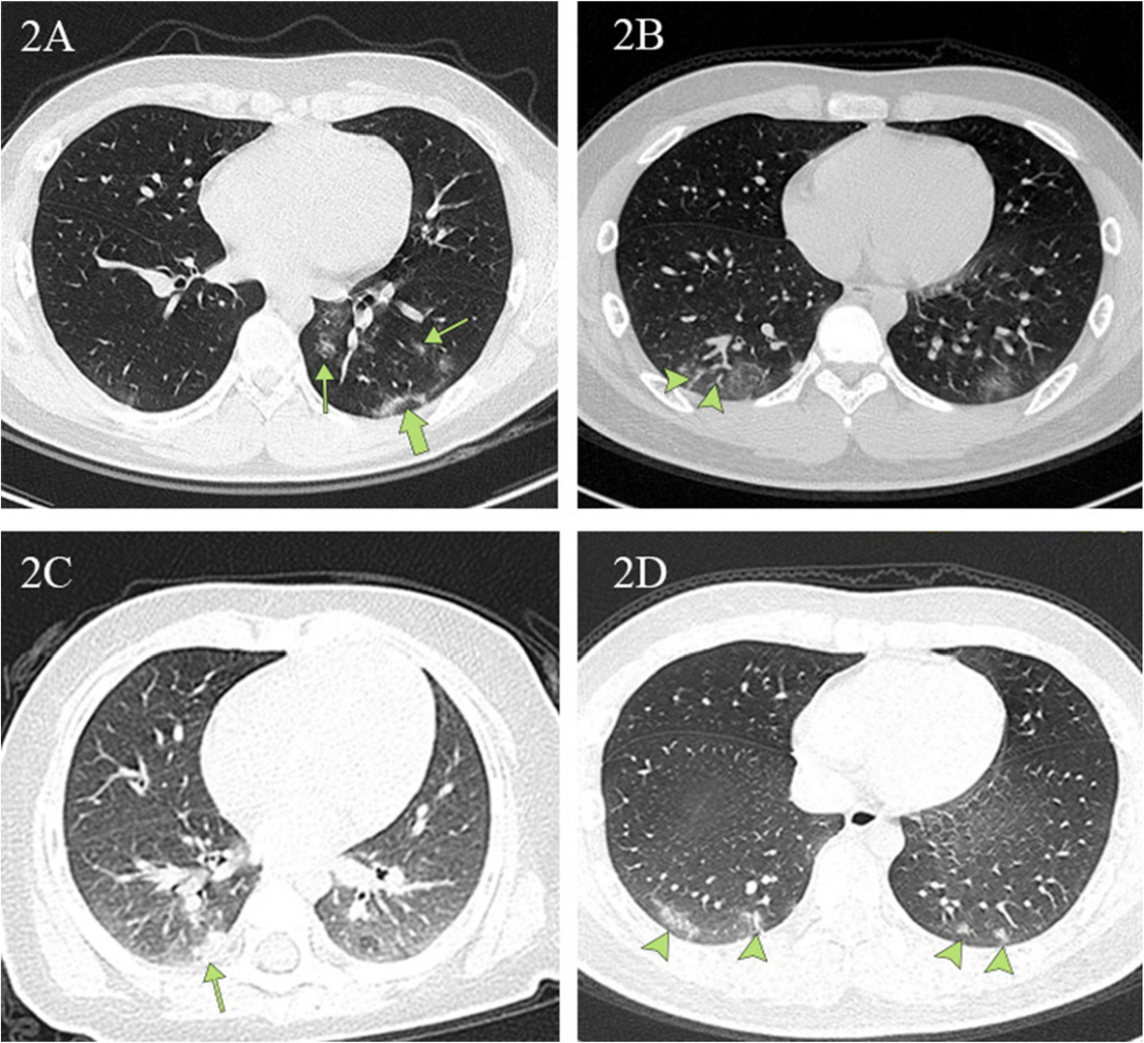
Chest CT images depicting typical radiographic findings of COVID-19 pneumonia in children. 2A A unilateral chest CT from a 14-year-old boy with a cough. Ground-glass opacities under and parallel to the pleura (thick green arrow) in the inferior lobes of the left lungs. Ground-glass opacities distributed along the bronchovascular bundle (thin green arrow). 2B Bilateral ground-glass opacities with vascular thickening (arrowheads) in the subpleural area from a 13-year-old boy with a fever and a cough. 2C Local patchy shadowing (green arrow) image from a 6 month-old girl with a fever and a cough. 2D Lesions in the lower lobe of both lungs (green arrows) on chest CT obtained from a 15-year-old boy with a fever and a cough
